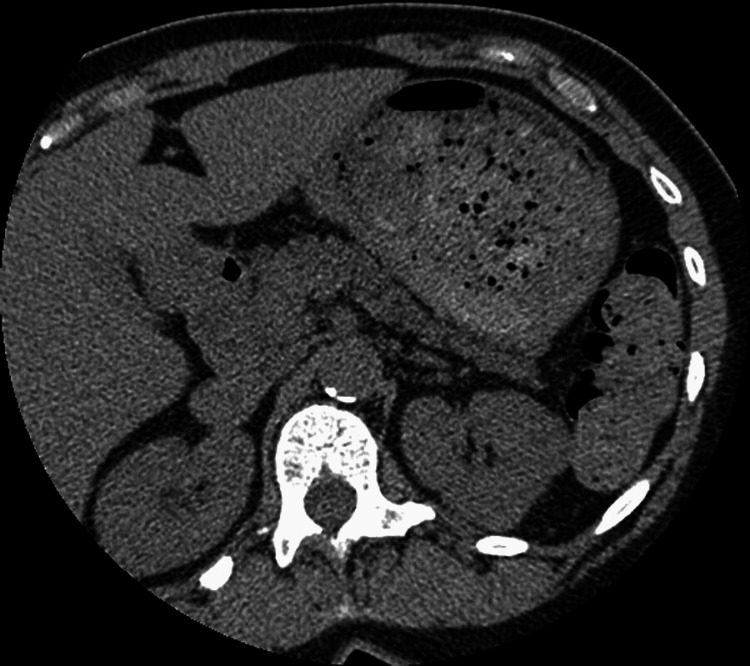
Cardiac CT examination from a year prior demonstrating a normal non-contrast appearance of the kidneys and retroperitoneum.
